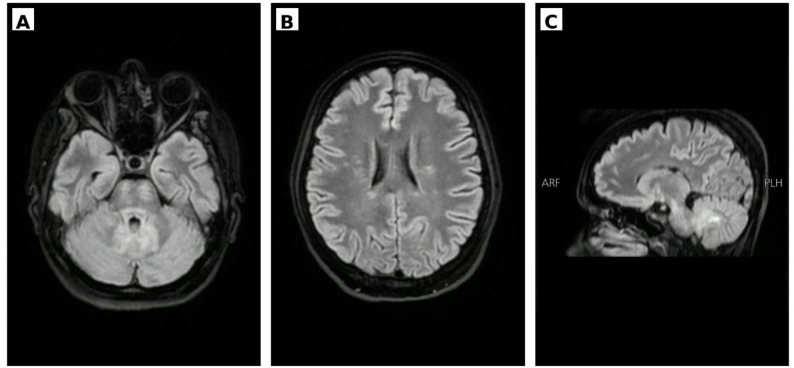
A (Axial T2 FLAIR – infratentorial level): Axial T2 fluid-attenuated inversion recovery (FLAIR) image at the level of the brainstem demonstrating hyperintense demyelinating lesions involving the pons and cerebellar white matter, consistent with infratentorial involvement. B (Axial T2 FLAIR – supratentorial level): Axial T2 fluid-attenuated inversion recovery (FLAIR) showing multiple bilateral hyperintense lesions in the periventricular and subcortical white matter, in keeping with an demyelinating process. C (Sagittal T2 FLAIR): Sagittal T2 fluid-attenuated inversion recovery (FLAIR) image demonstrating hyperintense white-matter lesions involving the periventricular region and posterior fossa, supporting the diagnosis of central nervous system demyelination
